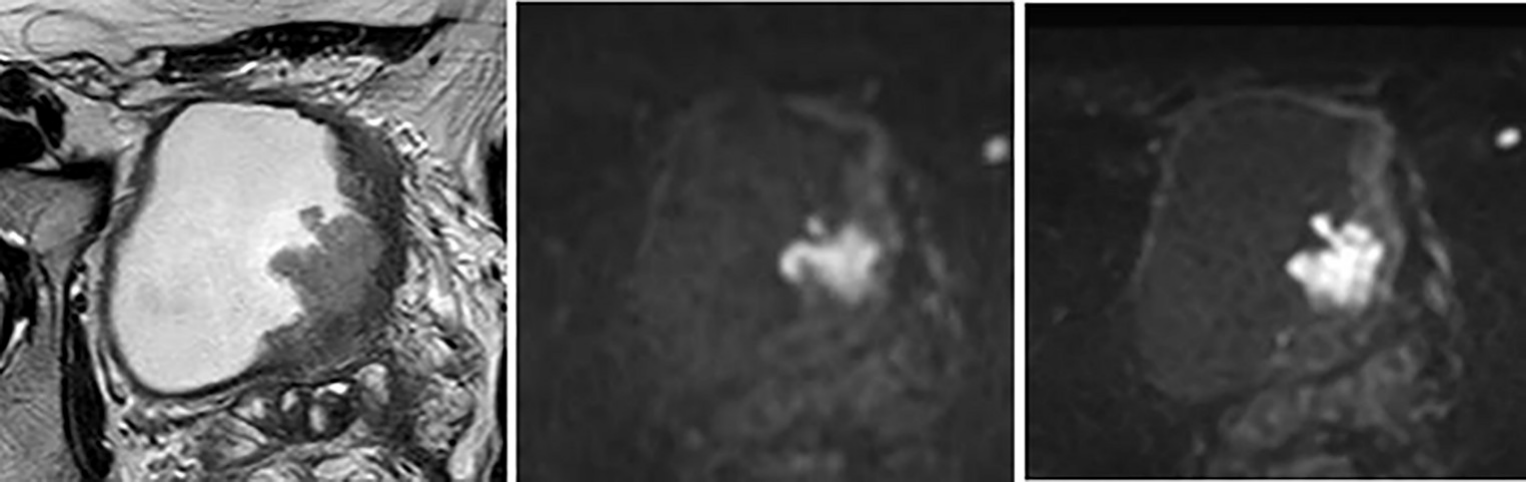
66-year-old man with the urothelial carcinoma of pT2 on the anterior wall of the bladder. (A) T2WI (B) fFOV DWI (C) Zoomed DWI. The tumor appeared as a lesion without stalk in both fFOV and Zoomed DWI, and score of VI-RADS was 3 for both fFOV and Zoomed DWI series.
